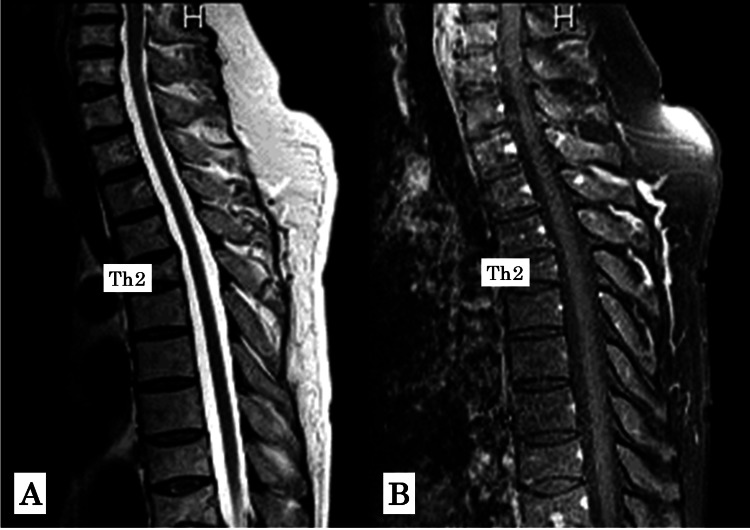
Thoracic spine MRI at three months after treatment initiation. (A) T2-weighted sagittal image showing normalization of signal intensity. (B) Gadolinium-enhanced sagittal image demonstrating resolution of contrast enhancement.
